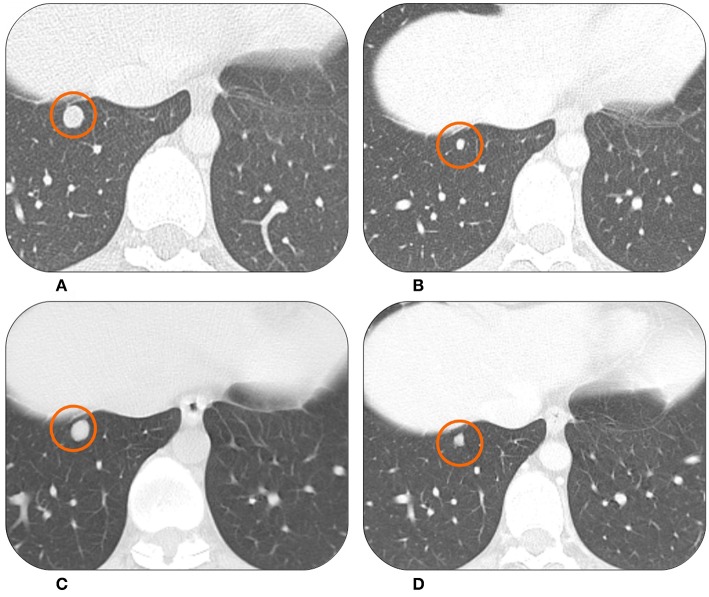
The CT scan of this patient showed lung metastases from synovial sarcoma at the baseline in February 2015 (A). At the first radiological evaluation in April 2015 there was a partial response (B). The patient was on drug holiday from June 2016 to April 2018 when the same lesion started to grow again (C). It then showed a good response to Trabectedin re-challenge in July 2018 (D).
